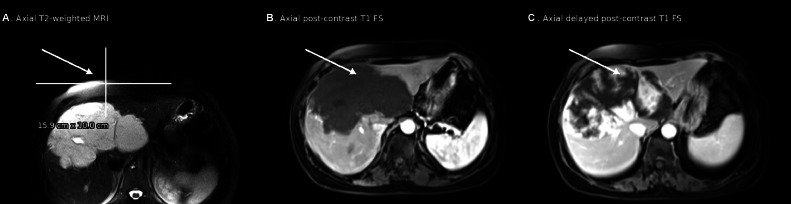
Follow-up MRI examination (August 2023). (A) Axial T2-weighted image demonstrates mild interval enlargement while preserving typical T2 hyperintensity; white arrows indicate the lesion and measurement lines show transverse and anteroposterior dimensions of 15.9 × 10.0 cm. (B) Axial postcontrast T1-weighted image again demonstrates peripheral nodular enhancement. (C) Axial delayed post-contrast T1-weighted image demonstrates persistent progressive centripetal enhancement without suspicious washout.
